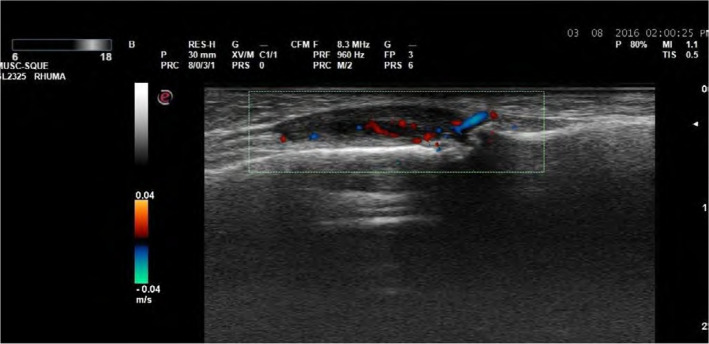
Ultrasonography of the second left intercarpal joint showing positive Doppler synovitis (grade 3).
